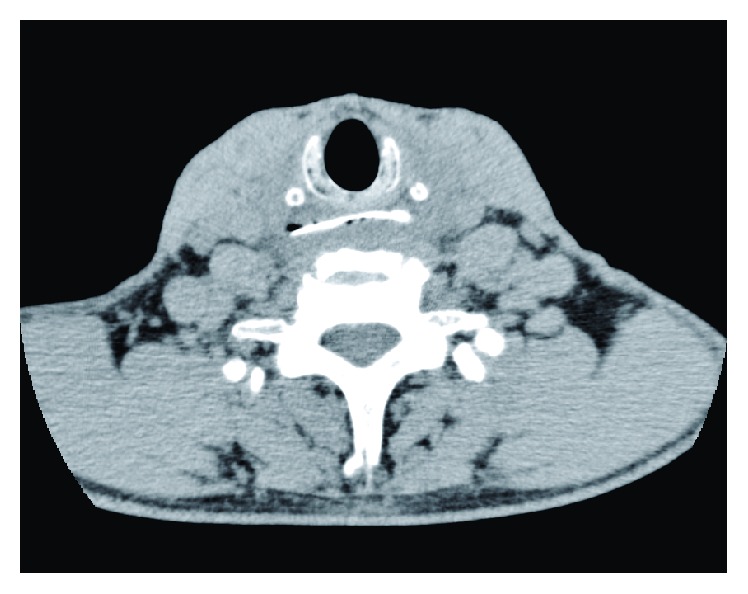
Noncontrast axial computed tomography showing a fish bone measuring 37 mm in length, lodged horizontally at the level of the lower end of the cricoid cartilage. No findings suggestive of mucosal perforation or soft tissue edema around the esophagus are apparent.
